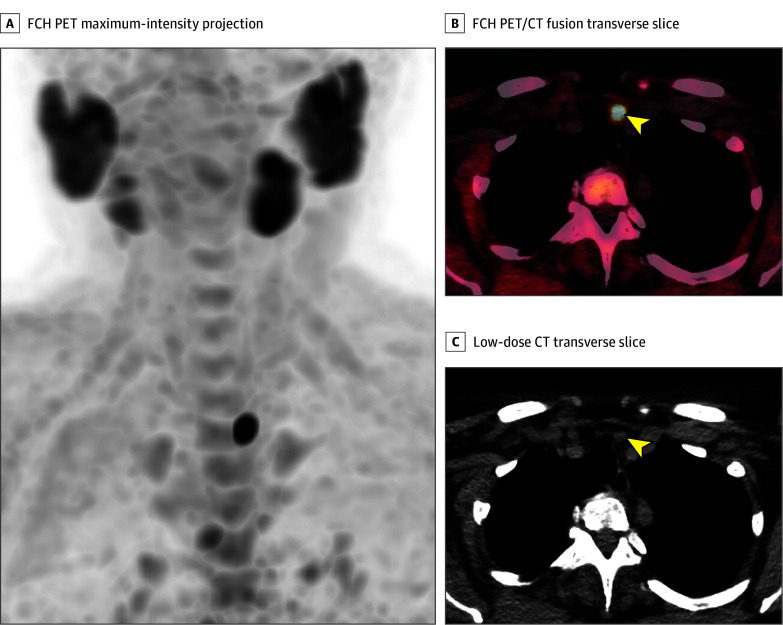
First-Line F18-Choline (FCH) Positron Emission Tomography (PET)/Computed Tomography (CT) Images of a Patient With Primary Hyperparathyroidism (PHPT) A, FCH PET maximum-intensity projection; B, FCH PET/CT fusion transverse slice; and C, low-dose CT transverse slice, showing high FCH uptake in an 11-mm left inferior PTA (arrowheads). The patient underwent minimally invasive parathyroidectomy, leading to normocalcemia during follow-up. No complications occurred.
